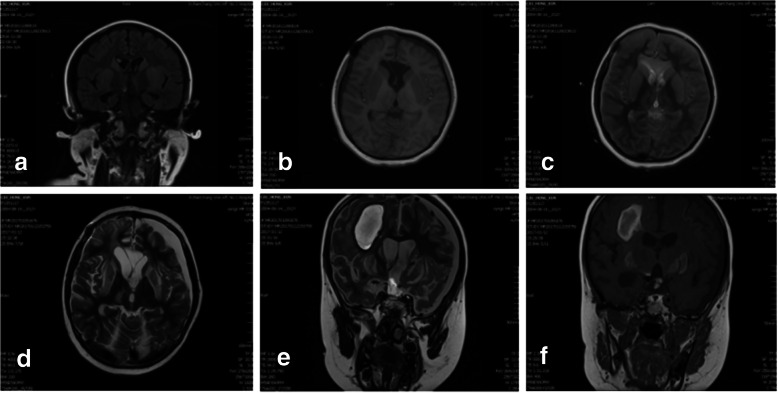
Postoperative day 16 and 30 MR images. a, b, c: The three images showed slightly symmetrical abnormal lesions at basal ganglia nucleus taken on postoperative day 16. Coronal FLAIR image (a) and axial T1-weighted MR image (b) show hypointense abnormality in the basal ganglia area. Axial T2-weighted MR image (c) showed slightly hyperintense change in the same areas. d, e, f: the three images were all taken on postoperative day 30. axial T2-weighted MR image (d) showed markedly symmetrical hyperintense abnormalities in caudate nucleus and lenticular nucleus; coronal T2-weighted (e) and T1-weighted (f) images showed a lesion in right frontal lobe with central hypointense and peripheral hyperintense signal, that was demyelination pseudotumor
